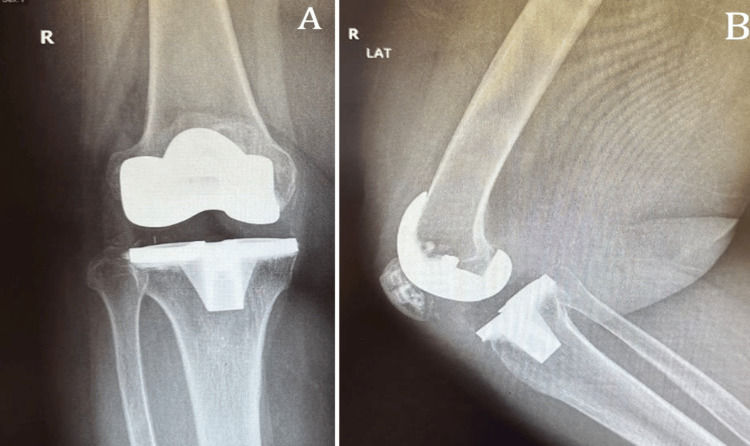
Right knee X-ray two months postoperatively: (A) AP view and (B) lateral view AP: anteroposterior
